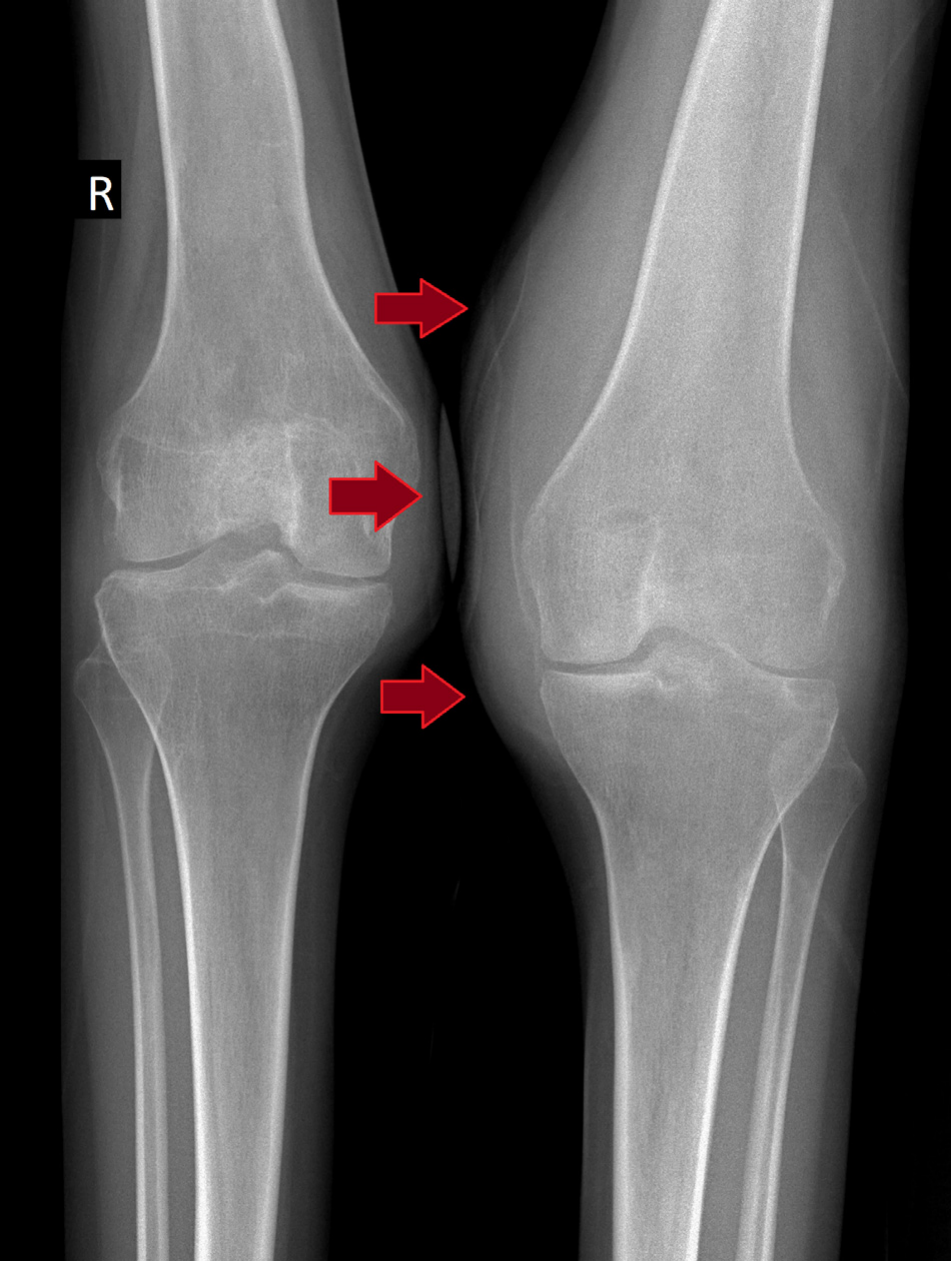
Anteroposterior X-ray showing a soft-tissue mass in the left knee. The arrows indicate the increased soft-tissue shadow in the left knee.
